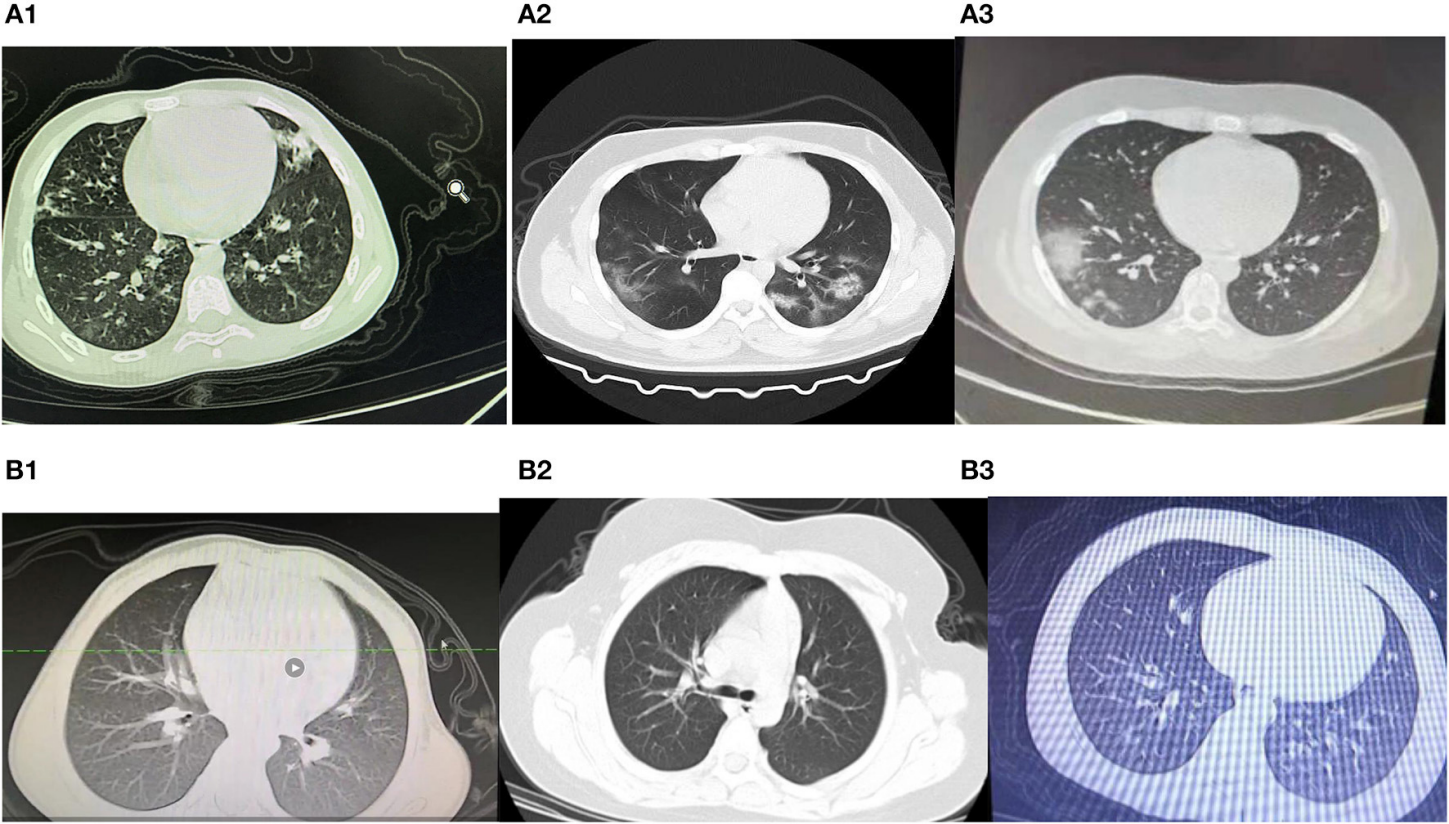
(A1–3) Chest CT image with typical abnormalities. (B1–3) Chest CT image with non-typical abnormalities.
